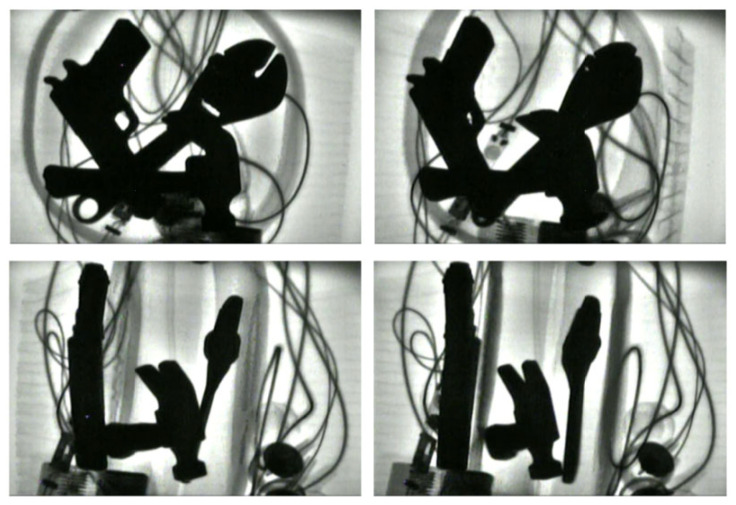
X-ray images with multiple prohibited items from different angles and complex background.
